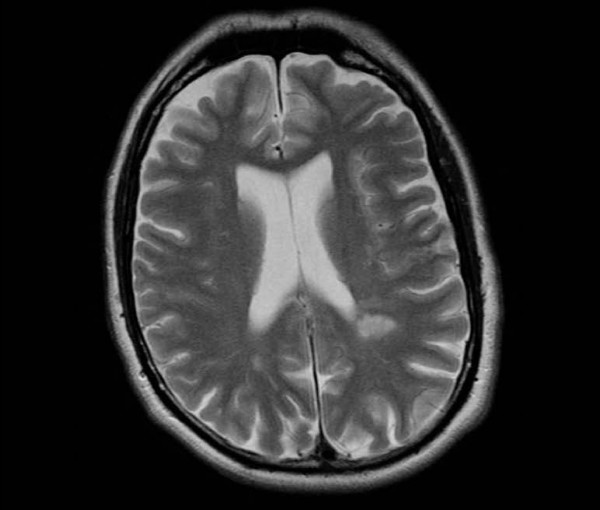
MRI of brain showing a large left parietal and multiple smaller cerebral infarcts.
